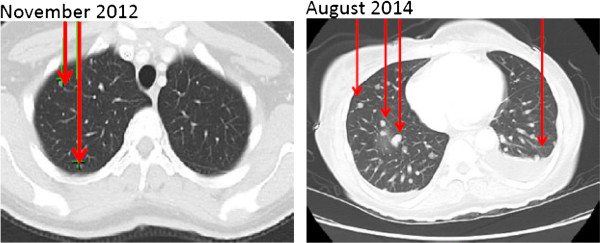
Computed tomography scan images demonstrating progression of metastatic colorectal cancer pulmonary disease indicated by arrows from the patient’s initial staging work up at our institution through her transition to aggressive comfort care.
